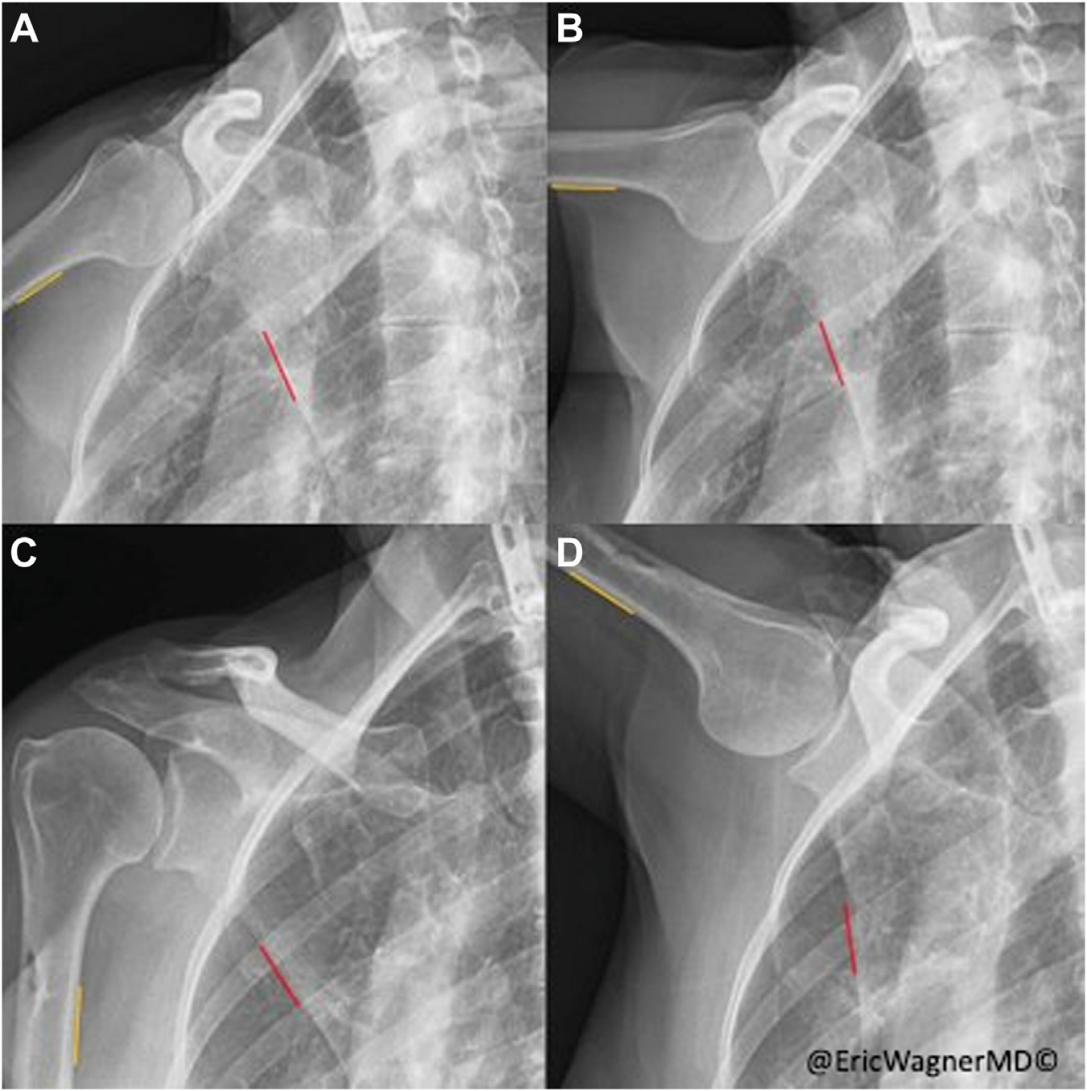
Clips taken from preoperative (A, B) and postoperative (C, D) DDRs displaying minimal (A, C) and maximal (B, D) angles of humeral abduction. Yellow lines indicate the zone used to measure humerothoacic angle. Red lines indicate the zone used to measure scapulothoracic angle. A and B show minimal to no change in scapulothoracic angle while C and D demonstrate a marked change in the scapulothoracic angle due to the restored external rotation of the scapula in D. *DDR*, dynamic digital radiography.
